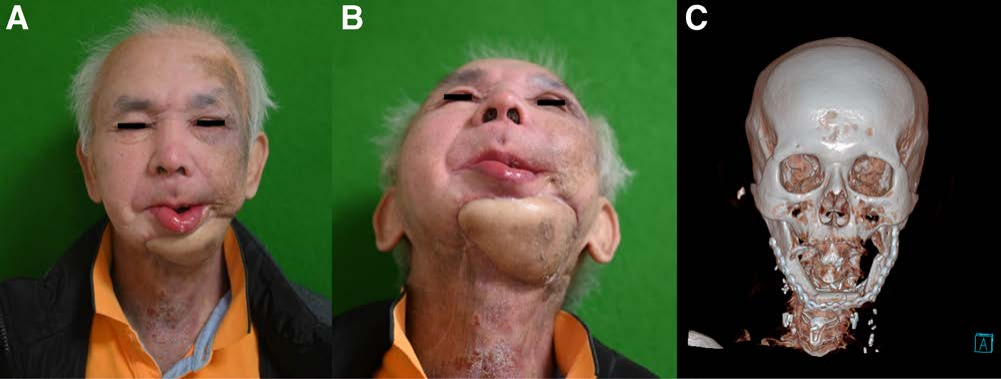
Patient at 6 months postoperatively. Excellent functional outcomes with good speech and swallowing and satisfactory aesthetic outcomes were achieved without flap bulkiness, scar contracture, or cancer recurrence. (A) Front view. (B) Worm’s eye view. (C) Computed tomography. The customized titanium plate was well fixed with osseous segments of the fibula free flap.
